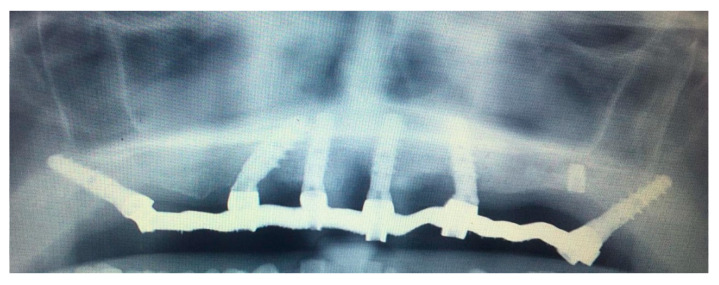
Digital panoramic X-ray showing the correct insertion of the six planned implants and the fit of the temporary prosthesis.
